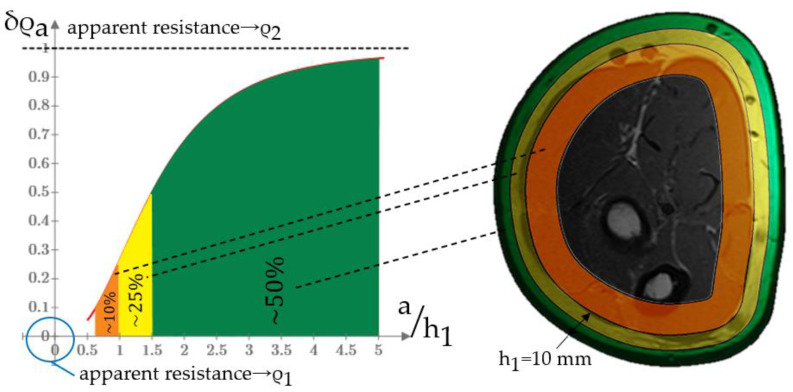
Relationship between the AR relative change and the ratio of the first layer thickness to the interelectrode distance (left) and its projection on the volunteer’s forearm MRI (girth 0.35 m) for ES with an interelectrode distance 0.01 m (right).
